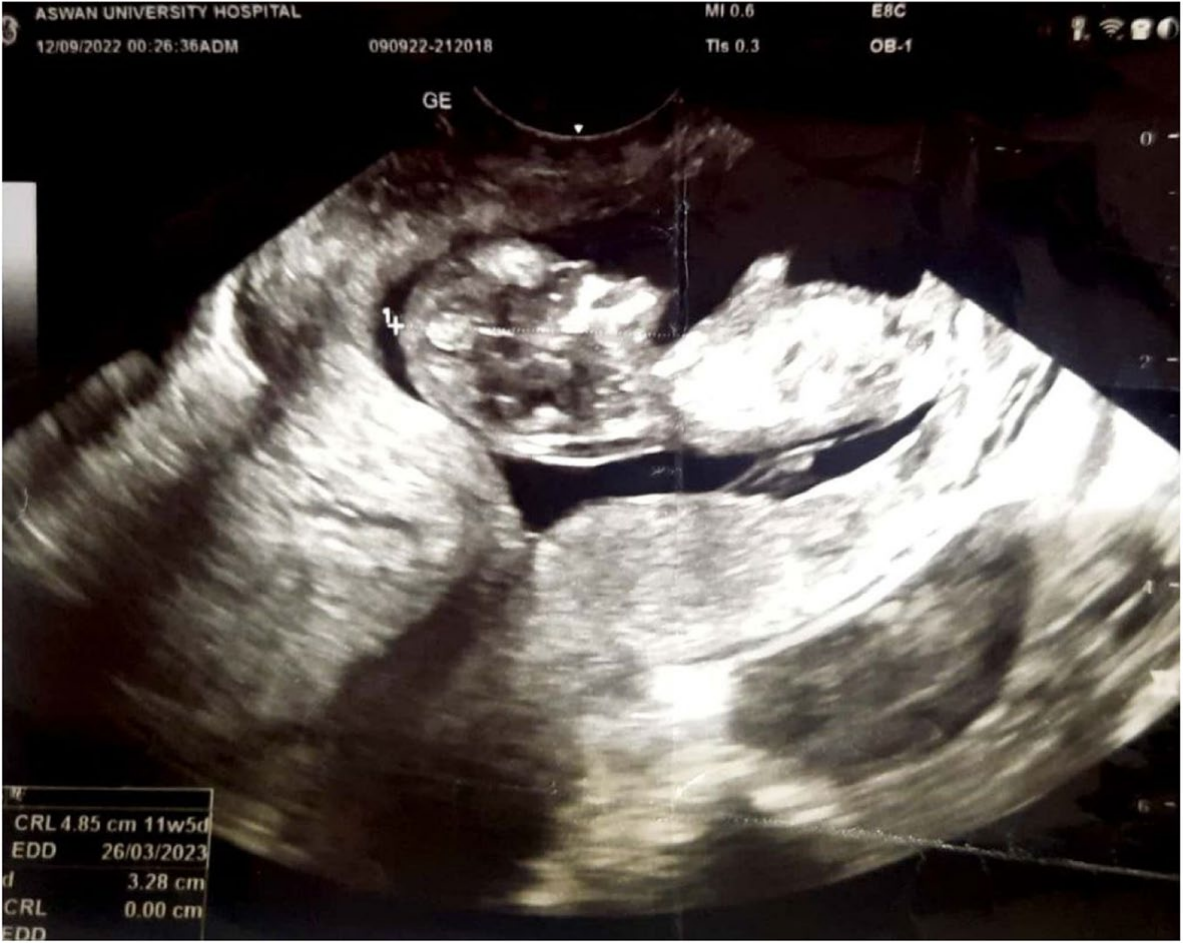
Transvaginal ultrasound on day 0 showing cervical ectopic pregnancy with crown-rump length measuring 48.5 mm, corresponding to 11 + 5 weeks
